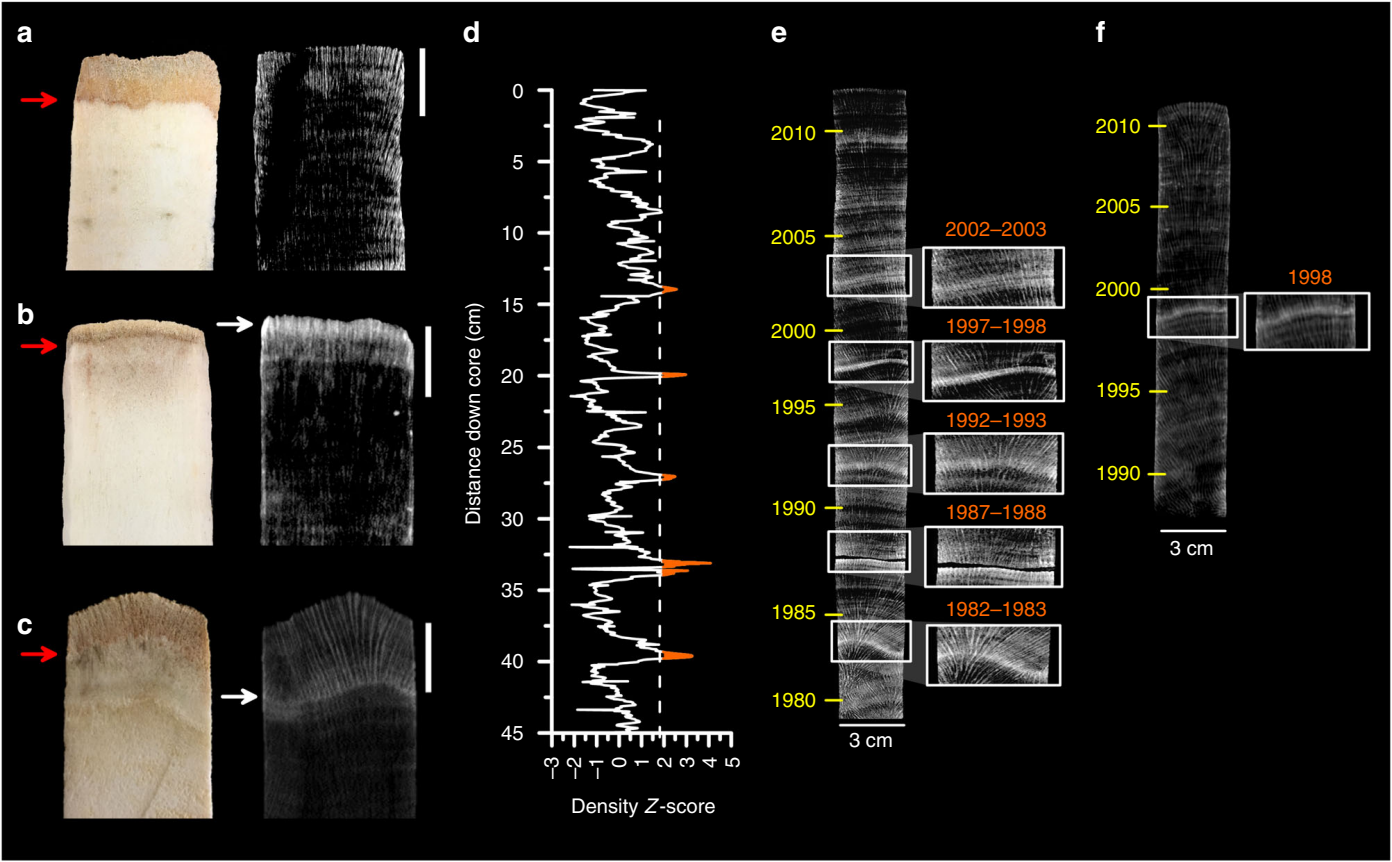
The skeletons of *Porites* corals record the 2015 bleaching (a–c) and historical bleaching events (d–e) that occurred on Jarvis Island, and for comparison, on Palau in 1998 (f). In the CT scan images, white is high-density, black is low density. In (a) CT scans of the tops of skeletal cores extracted from massive Jarvis *Porites* in 2012 show no sign of stress band formation. 2012 was an El Niño–Southern Oscillation neutral year and average tissue thickness measured in the core tops (left, red arrow) approached 1 cm, indicating the corals were energetically replete. Conversely, in (b), CT scans of 88% of the cores extracted in November 2015 revealed high-density stress band in the process of formation (white arrow). Tissue thickness of bleached colonies was reduced by ~75% (red arrow) indicating starvation. In (c), a core removed in April 2017 reveals recovery of tissue thickness (red arrow) and the 2015 stress band sequestered beneath new skeletal growth (scale bar = 1 cm). Stress bands are confirmed by automated analysis of density variations (d) where Z-scores > 2 (dashed line) in the detrended density time series are considered stress bands. Long *Porites* cores from Jarvis reveal multiple historical stress bands (e) whereas those from Palau have one or two, consistent with documented bleaching events on Palau in 1998 and 2010 (f)
